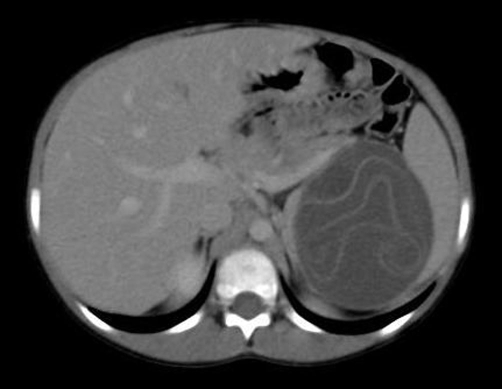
CT scan demonstrating floating membrane in the splenic cyst and multiple cysts in the peritoneal cavity.
